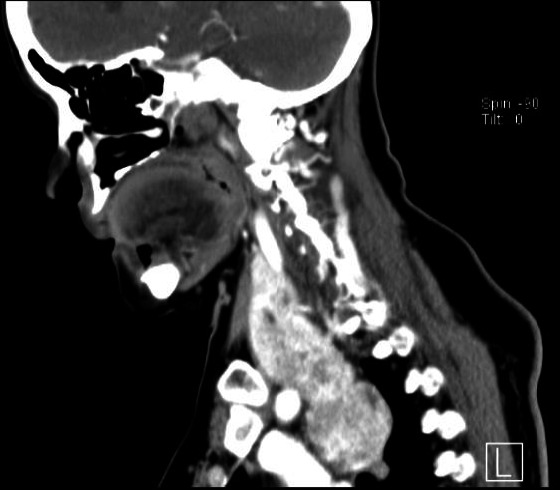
Sagittal section of the CT scan
